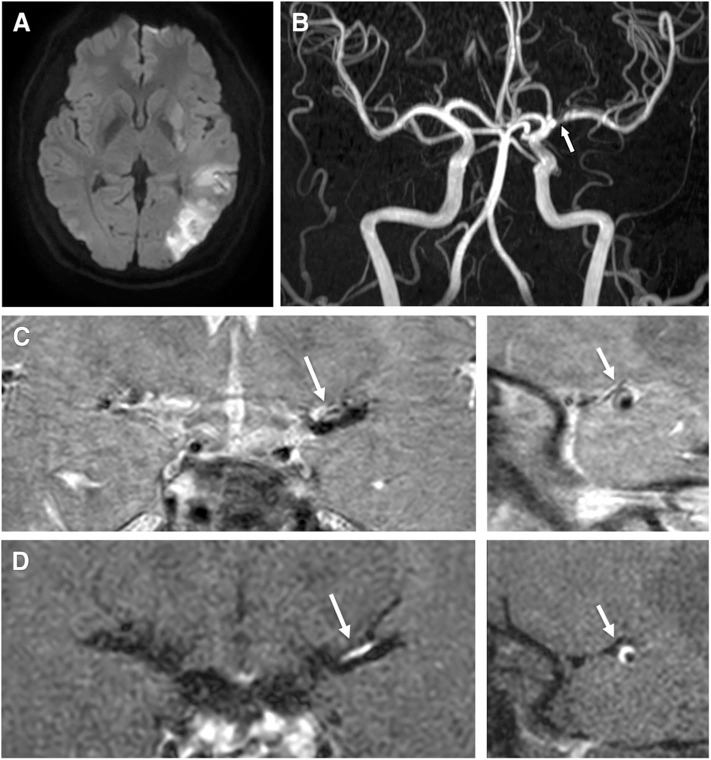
A 40-year-old male patient presented with right hemiparesis and aphasia with low-density cholesterol, total cholesterol, and Apo (b) levels of 178 mmol/L, 225 mmol/L, and 137 g/L, respectively. (A) Axial DWI shows a high signal intensity lesion in the left parietal region. (B) TOF-MRA shows severe stenosis in the left middle cerebral artery (proximal M1, arrow). (C) Pre-contrast 3-dimensional proton-density black-blood magnetic resonance images. (D) Post-contrast images (left: coronal plane, right: sagittal plane) show eccentric wall thickening (arrow) with enhancement.
